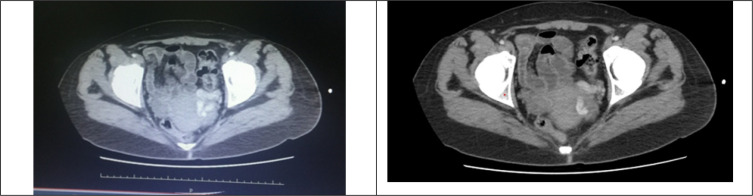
Axial view of intravenous contrast-enhanced abdominopelvic computed tomography scan of the patient
